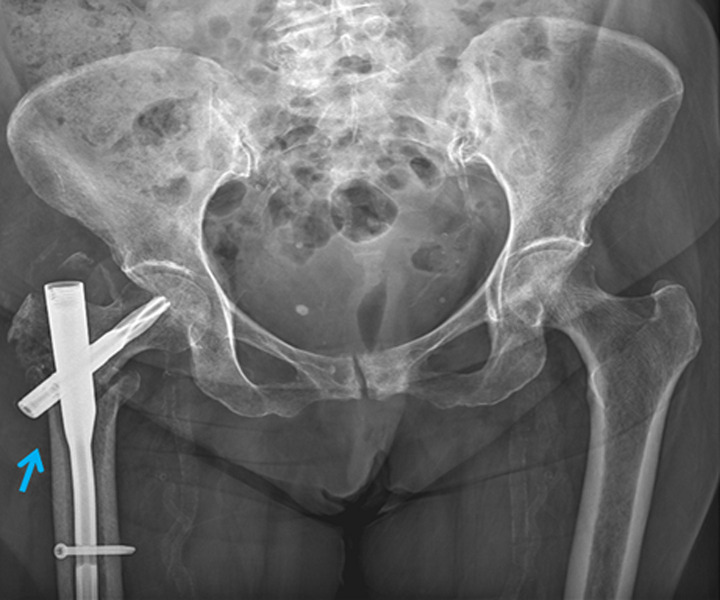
postoperative X-ray of pelvis at 6 months follow-up showing proximal femoral nail (PFN A2) on right side
